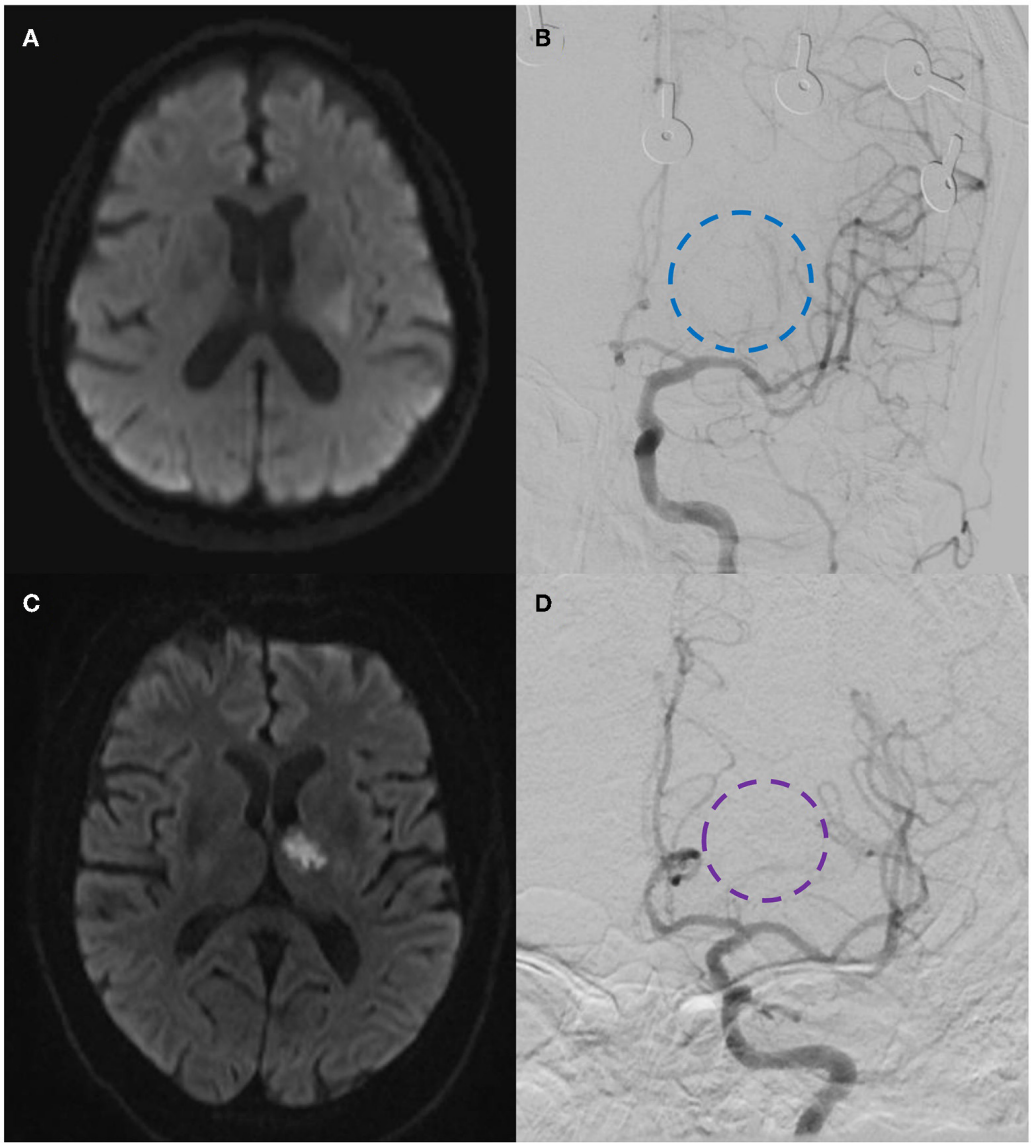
Patient 1: (A) Diffusion-weighted magnetic resonance imaging (DW-MRI) showing a subtle hyperintense signal in the left internal capsule and thalamus. (B) DSA showing hypoperfusion of the medial and lateral lenticulostriate penetrating arteries (dashed-blue circle). Patient 2: (C) DW-MRI showing a hyperintense signal in the left thalamus and internal capsule. (D) DSA showing a decreased vascular perfusion in the lenticulostriate penetrating arteries of the left middle cerebral artery (dashed-purple circle).
